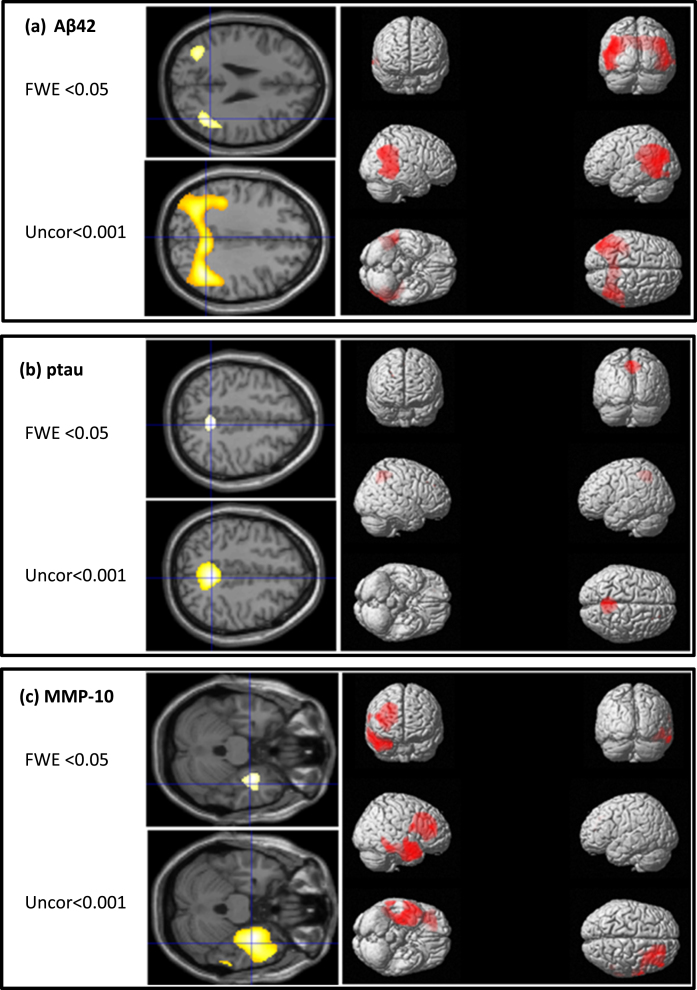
Overlays of clusters of hypoperfusion on MRI associated with changes in (a) Aβ_42_, (b) ptau, and (c) MMP-10 at FEW <0.05 and uncorrected <0.001 levels and surface rendering at the uncorrected <0.001 level.
